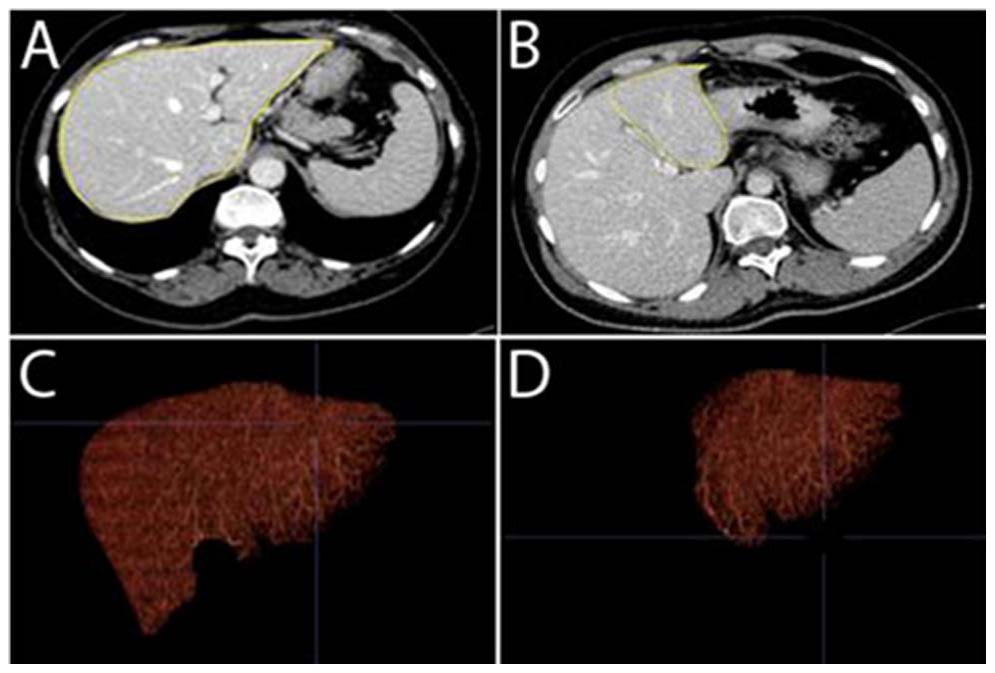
Manual Commercial Software (TR) – Image Example. A Transverse image of the portal-venous phase – manual outline of the entire liver (yellow). B Transverse image of the portal-venous phase – manual outline of liver segments II/III (yellow). C Volume rendering (coronal view) resulting after manual outline of the entire liver. D Volume rendering (coronal view) resulting after manual outline of liver segments II/III. Note: in each live liver donor, CT-volumetry of the entire liver as well as of the future liver graft (transplanted liver segments) were performed to ensure that the postoperative liver volume is adequate.
